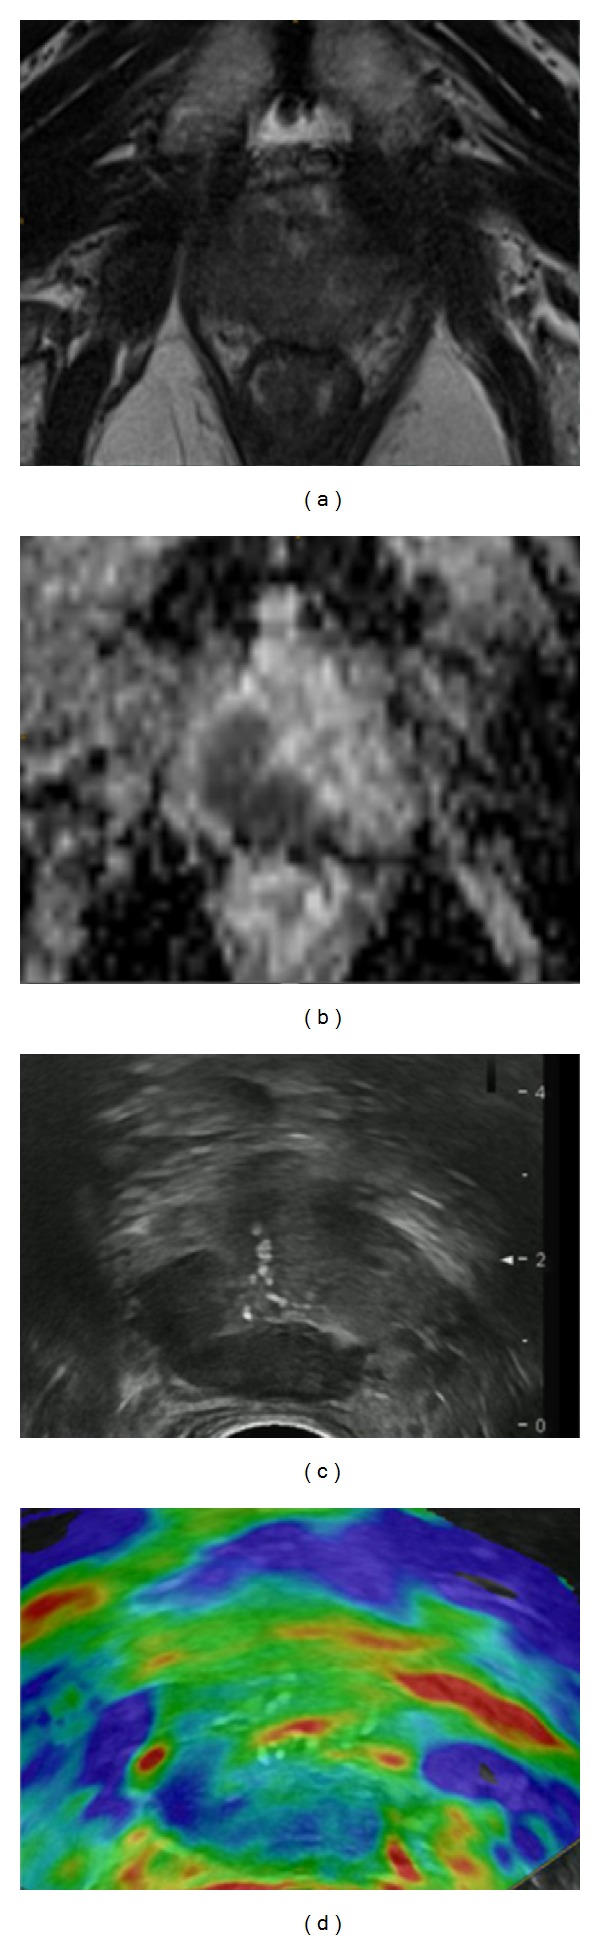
PCa (dorsal right peripheral zone) is shown on both modalities. (a) T2-weighted imaging showing the cancer as an area of decreased signal intensity; (b) apparent diffusion coefficient showing the cancer as an area of restricted diffusion; (c) grey-scale image showing the cancer as an area of decreased echogenicity; (d) elastogram showing the cancer as an area of decreased elasticity (colour-coded blue).
